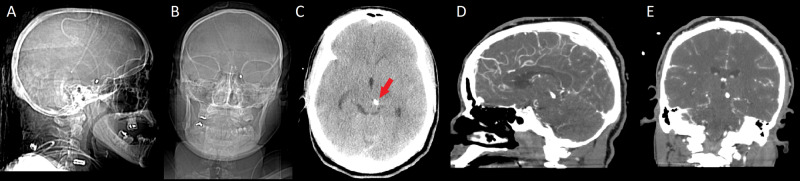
Sagittal scout (A), AP scout (B) Axial (C), Sagittal (D) and Coronal (E) CTA showing impingement of catheter tip on left tectum
